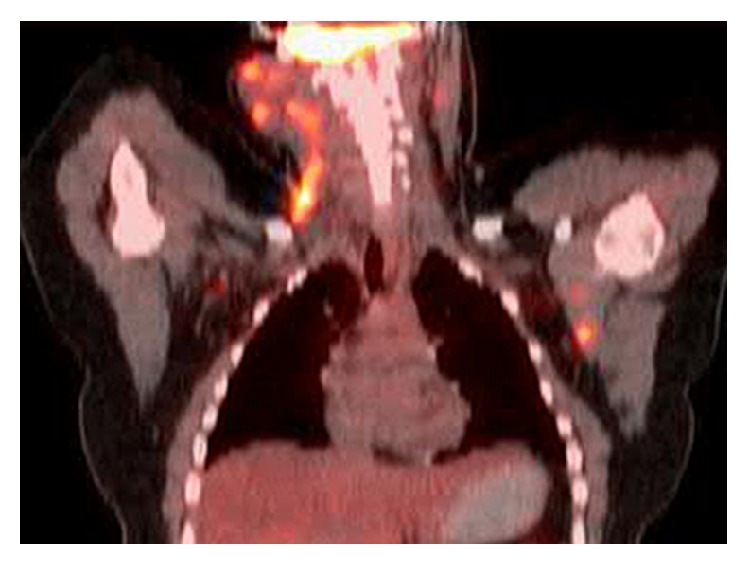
Positron Emission Tomography, Computed Tomography Scan. Fusion PET/CT demonstrating diffuse right cervical and left axillary lymphadenopathy.
